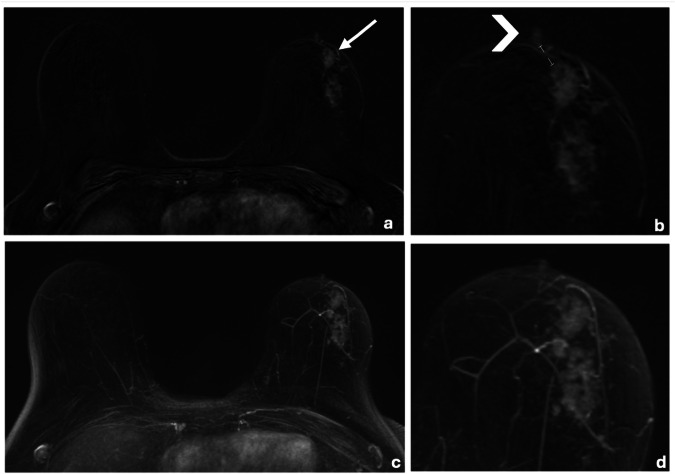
44-year-old woman with grade 3 invasive breast carcinoma of no special type (IBC-NST) with in situ component, undergoing preoperative breast MRI. The tumor was identified as multiple contiguous mass lesions located in the retroareolar region of the left breast (arrow) in the second post-contrast subtracted T1-weighted sequence (a). Three criteria suggestive of nipple-areolar complex (NAC) involvement were identified by both readers: (i) abnormal enhancement of the left NAC relative to the contralateral NAC (arrowheads) (b), (ii) a tumor-to-nipple (TTN) distance ≤ 10 mm (between calipers) (b) and (iii) nipple retraction (b). Contrast-enhanced T1-weighted MIP images confirmed and highlighted the described findings (c, d). Final surgical pathology examination after total mastectomy and axillary dissection confirmed multicentric grade 3 IBC-NST with an in situ component and NAC involvement
